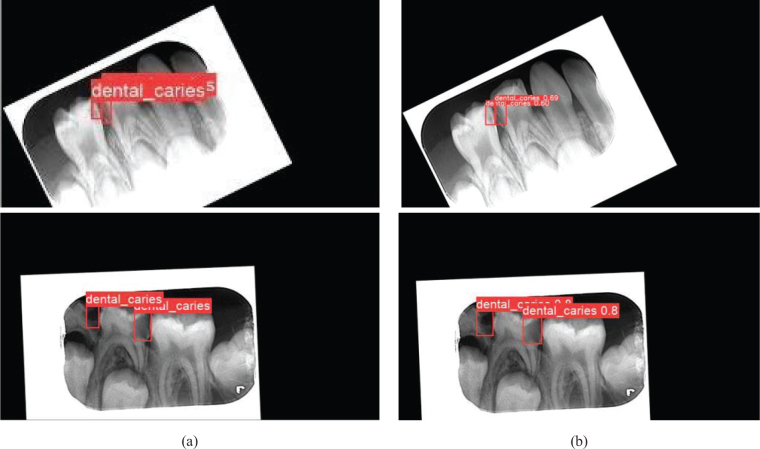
Evaluation of the detection performance of the proposed AI-based model with a comparison between labeling and testing stages on the same radiographic images. (a) Radiographic images labeled by the pediatric dentist before training and testing process and (b) the output images from the test.
